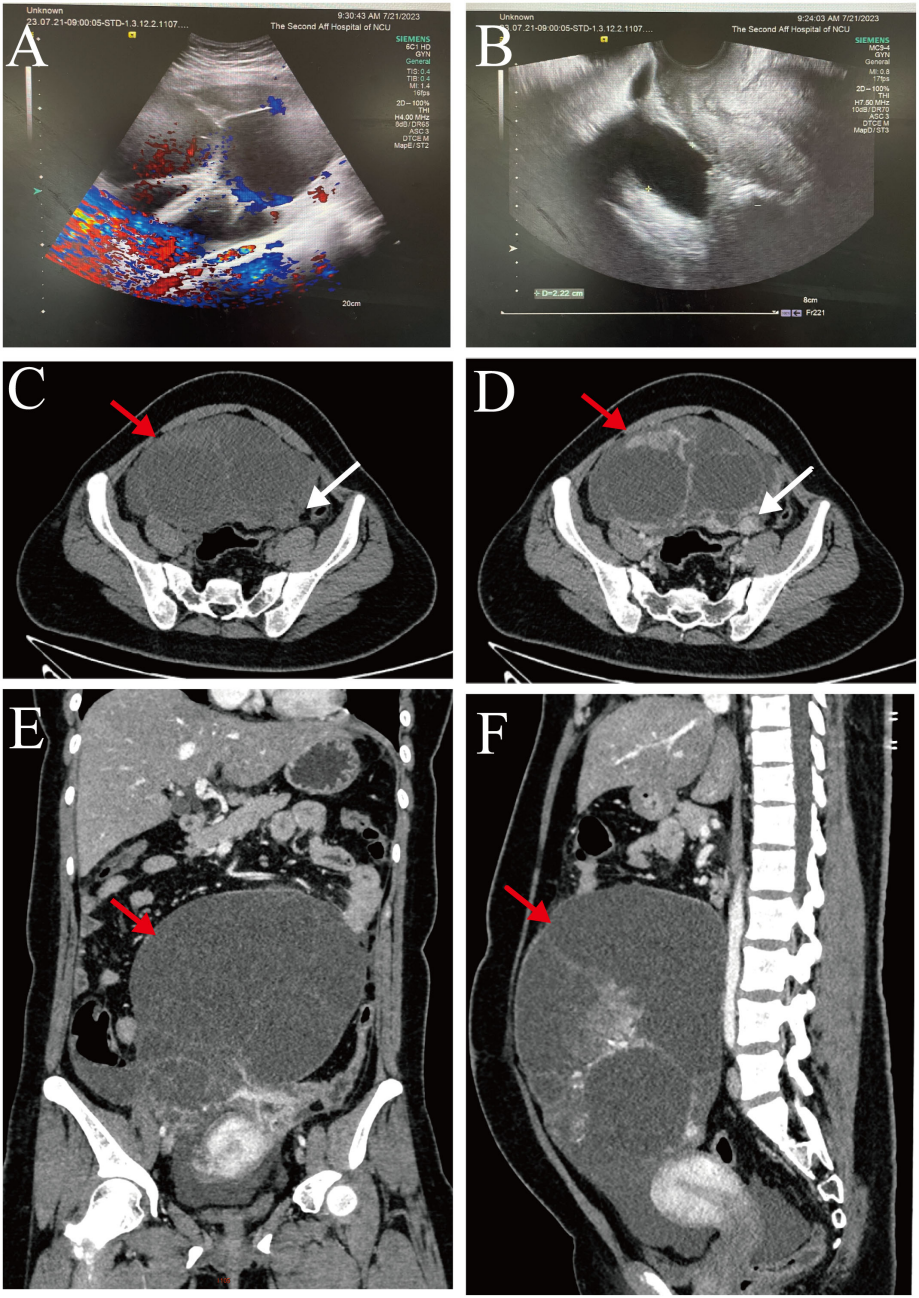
(A, B) Transvaginal color ultrasound scan: The left ovary showed unclear and there was a predominantly cystic mixed echogenic mass in the lower abdomen (size about 40 cm × 45 cm × 15 cm). (C-F) Whole-abdomen enhanced computed tomography images: There was a large cystic solid mass (red arrow) in the abdominopelvic cavity that was in close proximity to the left adnexa (white arrow).
